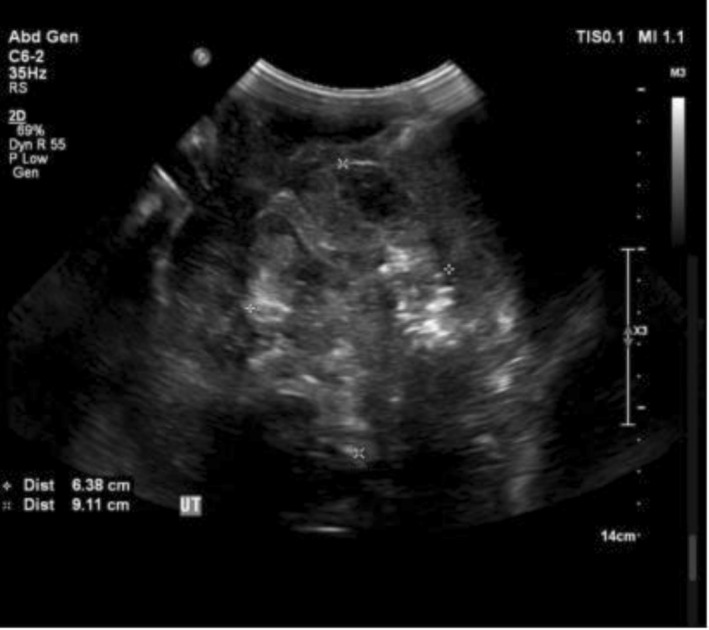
Ultrasonography showing a large echogenic abdominopelvic mass crossing the midline.
